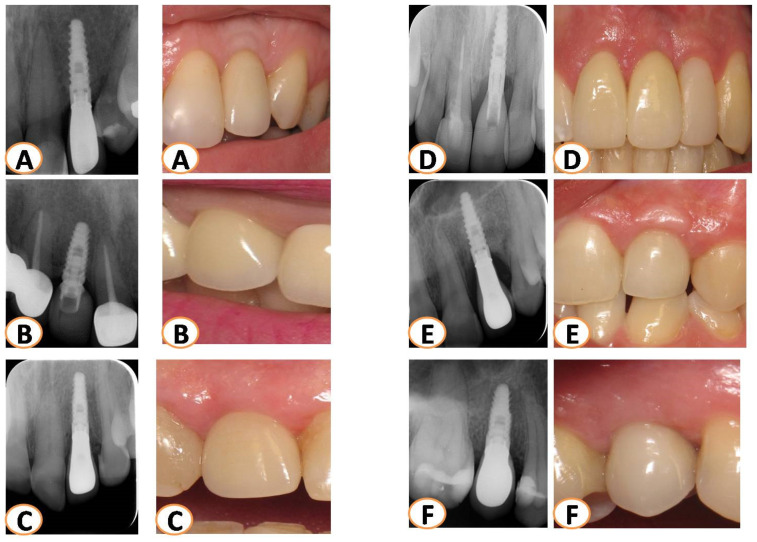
All patients were monitored for more than seven years with X-rays (parallel technique) and pictures. The last X-rays and photos taken were evaluated for each case. Several cases are presented in this figure (A–F).
